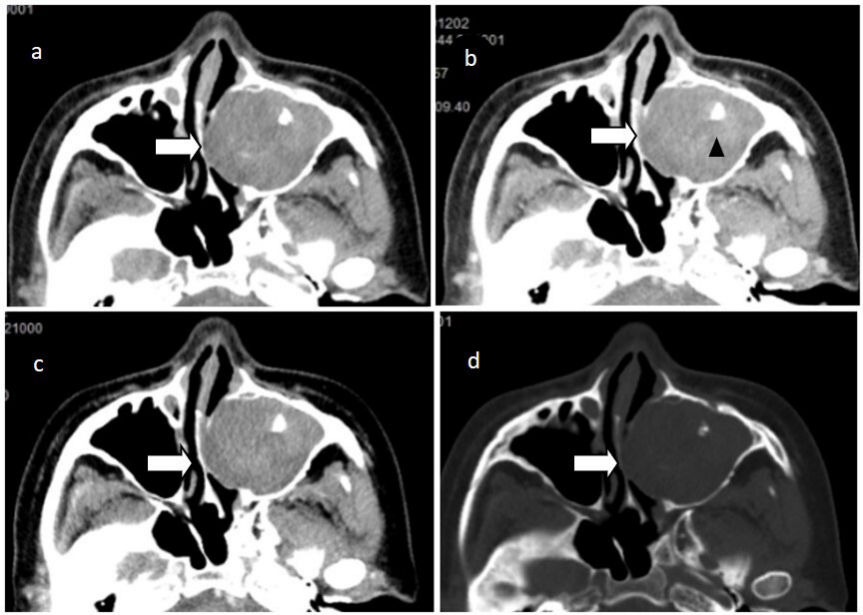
Axial non contrast-enhanced CT imaging(a) showed a round solid soft tissue mass(white arrows) in the left maxillary sinus with punctate calcification at its center(black arrow head). Contrast enhanced CT imaging(c and d) indicated mild uneven enhancement of the mass (an increase of about 5-10 HU); her maxillary sinus cavity was distended with bone resorption on its inner wall(d).
